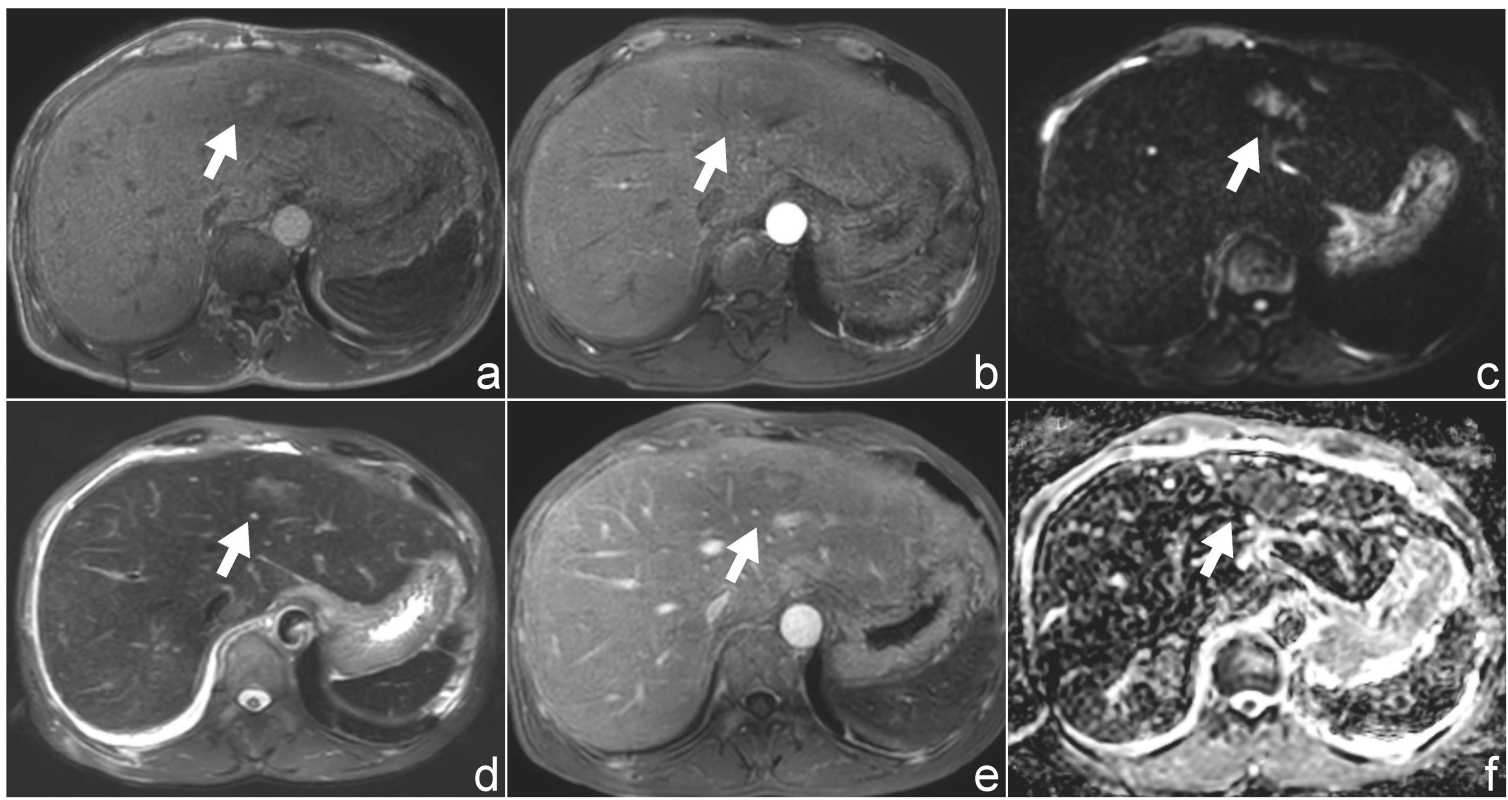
Magnetic resonance imaging (MRI) findings of the patient. (a, d) Axial T1-weighted (a) and T2-weighted (d) images demonstrate a hyperintense lesion in hepatic segment II. (b, e) Post-contrast T1-weighted images following gadolinium administration show minimal enhancement of the lesion. (c, f) Diffusion-weighted imaging (DWI, b = 800 s/mm²) and corresponding apparent diffusion coefficient (ADC) maps reveal mildly restricted diffusion.
